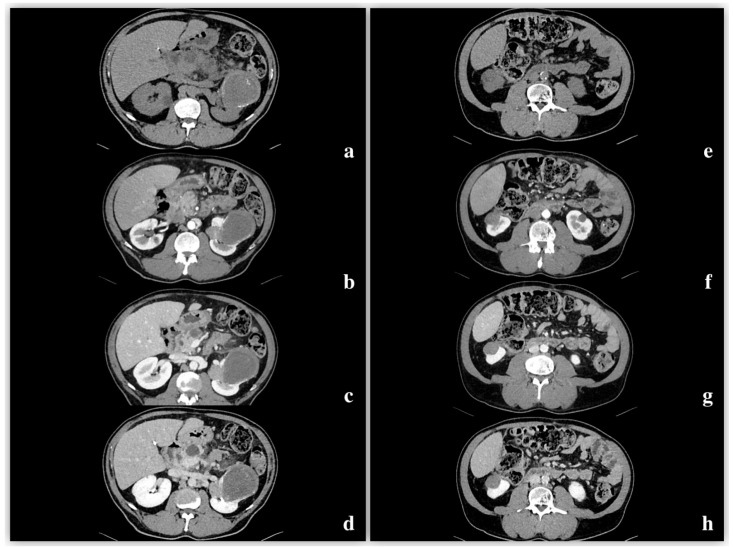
Patient 1 (right image) (e–h): On unenhanced CT, a slightly hypodense lesion (35 HU) with calcifications was detected along the anterior margin of the left kidney. During the corticomedullary phase (CMP), the lesion exhibited minimal enhancement (45 HU). In the venous phase, the lesion was increased to 68 HU, showing no significant washout but presenting hypodense areas within. In the excretory phase, there was no substantial washout except for a centrally located hypodense area (60 HU). Patient 2 (left image) (a–d): A papillary renal lesion located at the down pole of the right kidney is observed. Across all phases of the CT study, the lesion exhibits attenuation characteristics similar to those of a renal cyst, demonstrating a homogeneous, hypodense appearance with no significant enhancement or washout.
